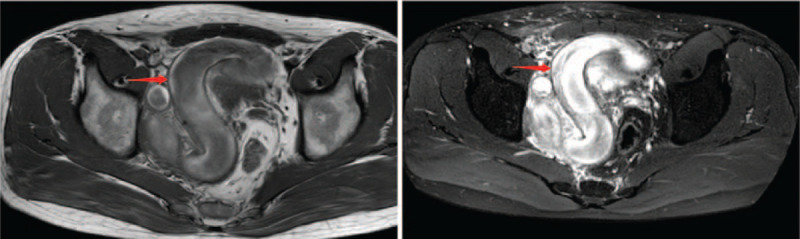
MRI scan of the pelvis showing a tortuous tube-shaped lesion with T1 and T2 signals of equal lengths on the right side of the pelvic cavity. A large amount of exudation can be observed around the lesion, and the boundary with the seminal vesicle gland on the right side is not clear. The enhanced scan shows the enhancement of the tube wall and its head end obturated to the right. The intermuscular space extends, and the prostate, rectum, and seminal vesicle glands are compressed and moved to the left.
